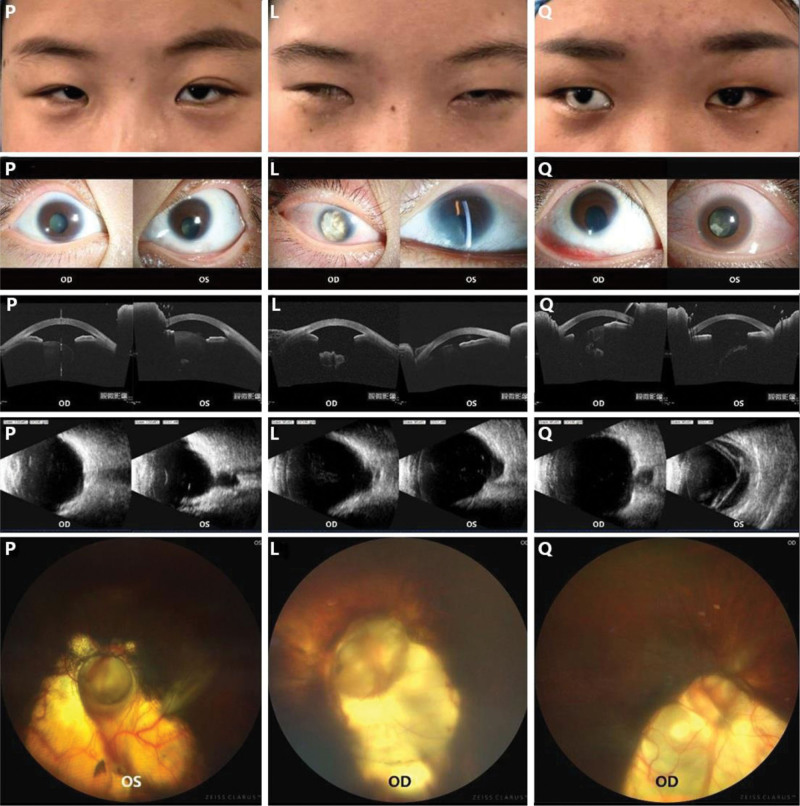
Clinical features of the 3 patients. Clinical pictures showed narrow palpebral fissures, bilateral ptosis accompanied by epicanthus and esotropia. Photographs of the anterior segments showed small cornea, iris coloboma and microspherophakia with uneven lens opacity. Anterior segment optical coherence tomography (AS-OCT) showed microspherophakia with uneven lens opacity, subluxation of lens and suspensory ligament relaxation. Ocular B-scan ultrasound indicated choroidal coloboma, optic disc coloboma and retinal detachment. Fundus photographs post cataract surgery showed optic disk coloboma and chorioretinal coloboma (P: the proband, L: patient L, Q: patient Q, OD: the right eye, OS: the left eye). AS-OCT = anterior segment optical coherence tomography.
